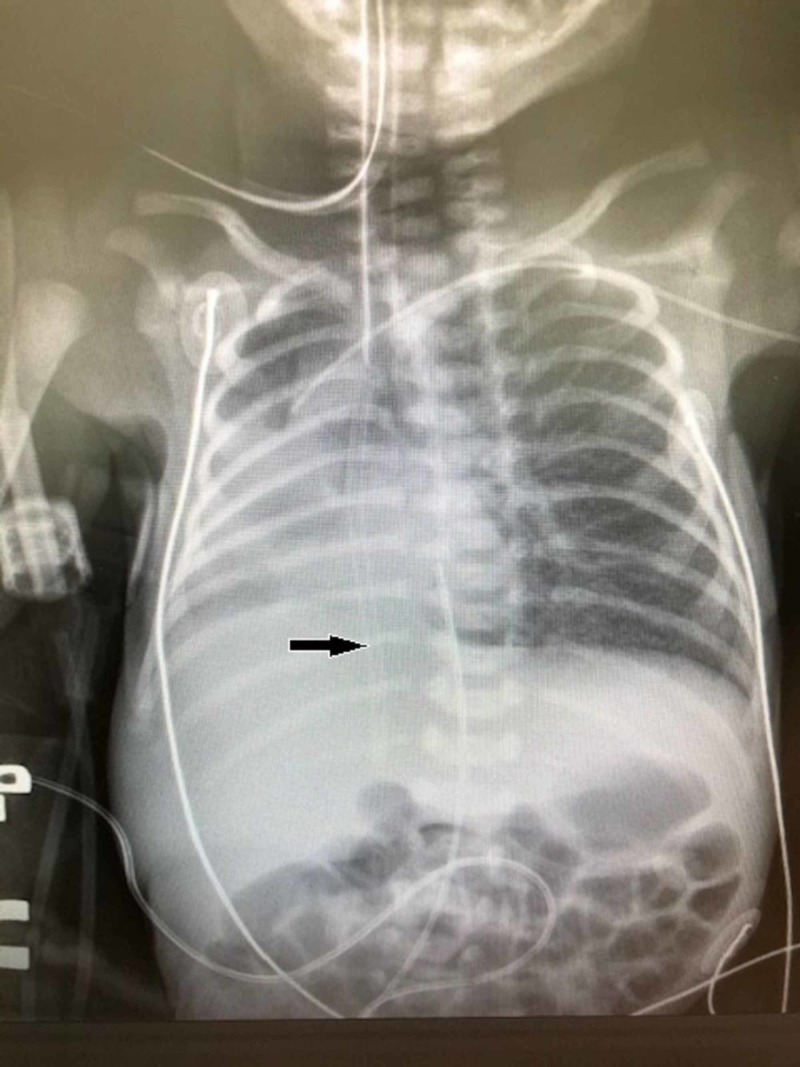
Chest X-ray of the patient obtained to verify PICC line placement The arrow notes the presence of a foreign body later found to be a portion of the plastic sheath covering the stylet used during intubation PICC: peripherally inserted central catheter
